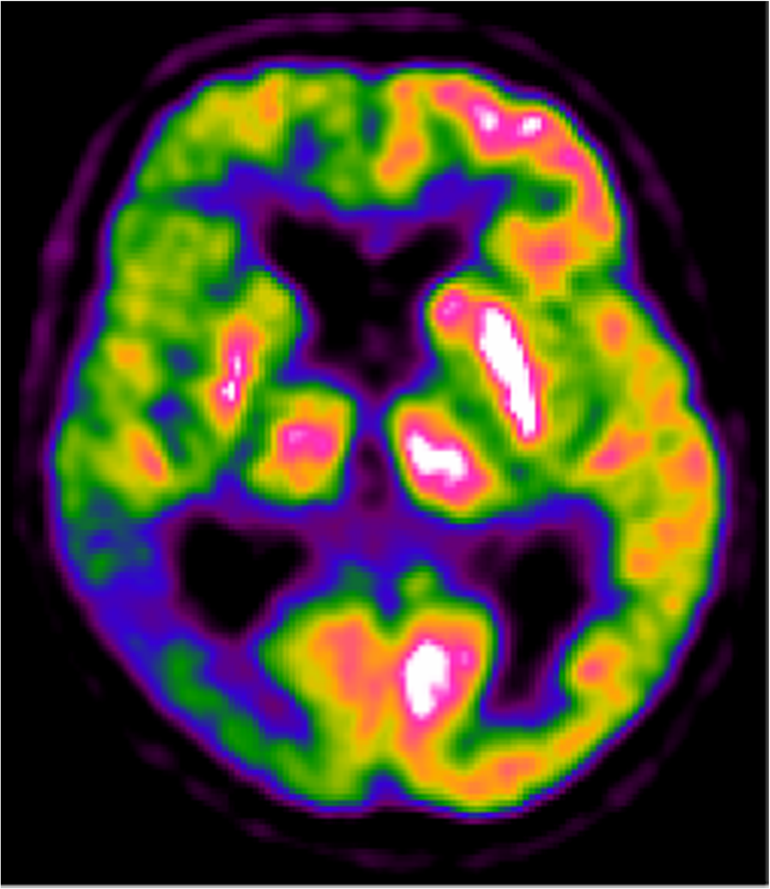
A 76-year-old patient with CBS and mild cognitive impairment (non-amnestic, multiple domain MCI; MMSE score 28/30). The FDG PET image shows severe hypometabolism in the whole right hemisphere, including the basal ganglia and thalamus, with more severe hypometabolism in the temporoparietal cortex. Dopamine transporter SPECT imaging showed severely reduced uptake in the right basal ganglia, suggesting CBD
